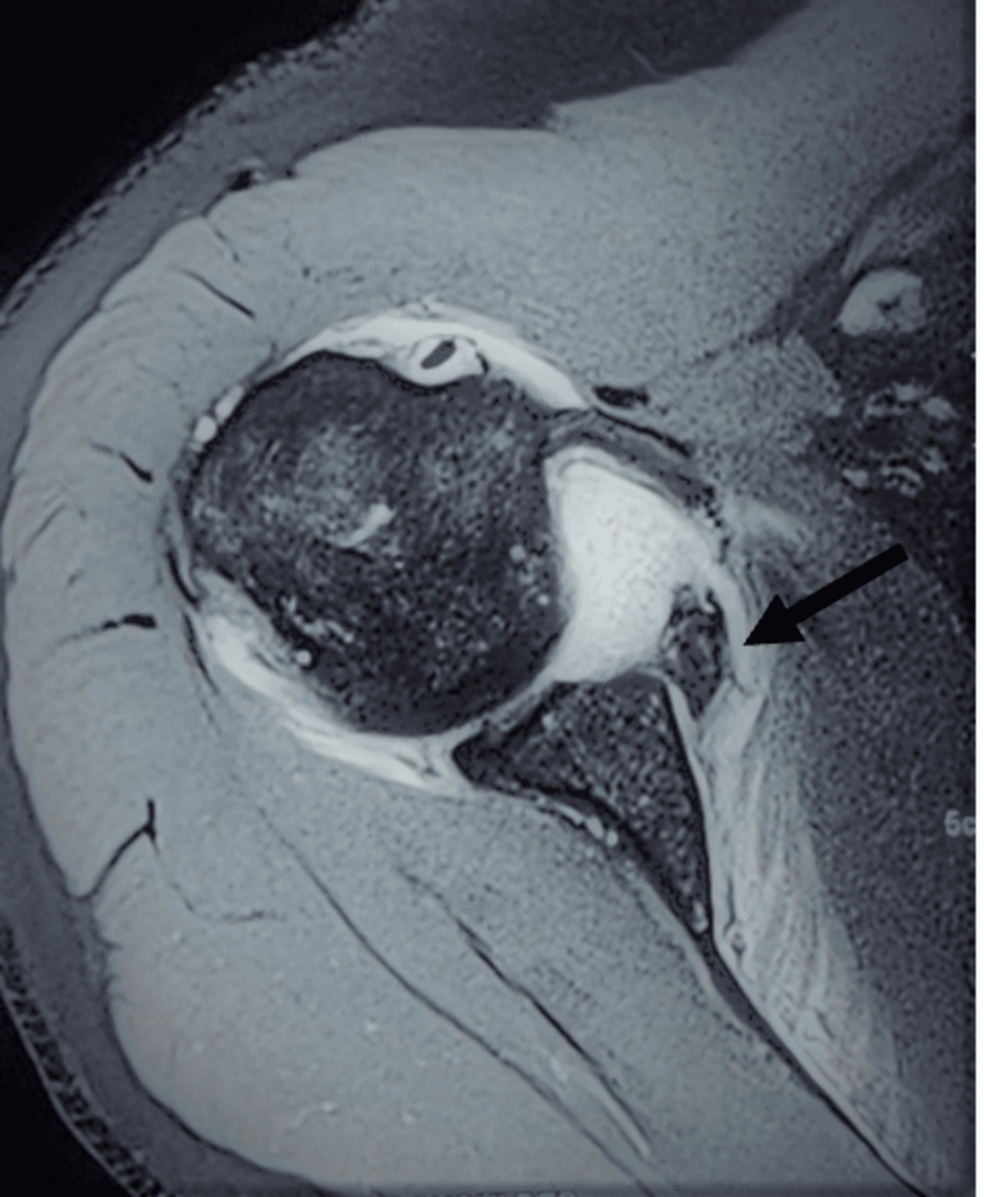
MRI showing a bony Bankart lesion with osseous fragment attaching to the labro capsular complex (indicated by the black arrow).
